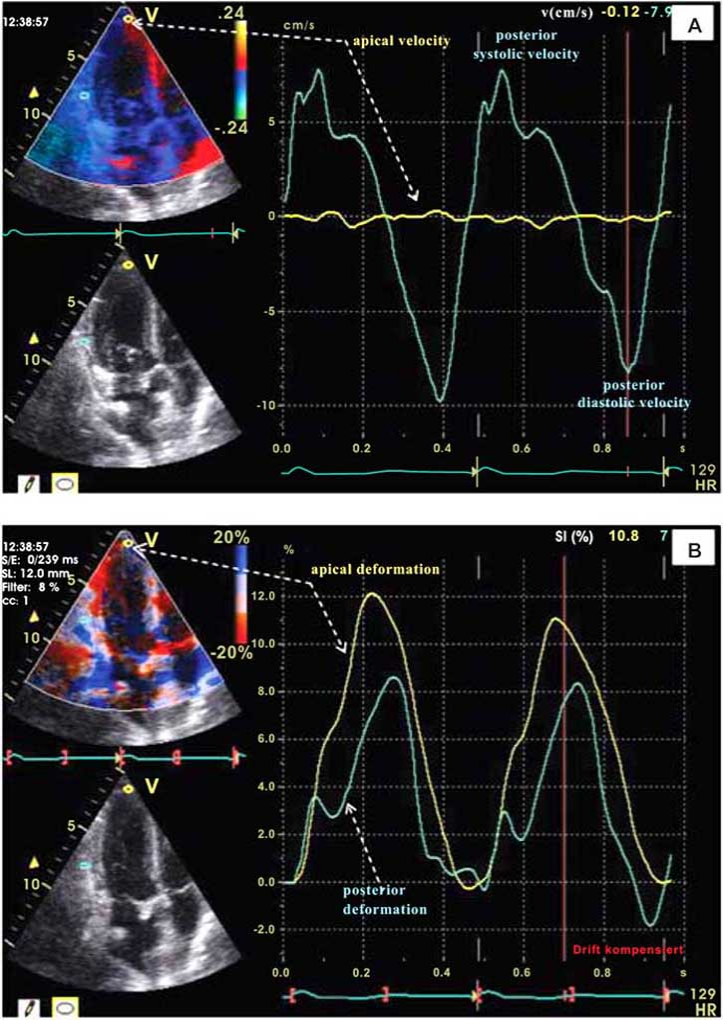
Tissue Doppler derived left ventricular wall motion velocity (panel A) and myocardial strain (panel B) images from a heart transplanted patient with normal cardiac function and no evidence of coronary artery disease. The velocity and strain curves were obtained in apical long axes views during the same cardiac cycle from the same two myocardial regions (posterior-basal and apical). Because of the velocity gradient which normally exists between the basal and apical LV regions (highest at the base and lowest in apical regions), the assessment of wall motion velocity is not useful for detection of regional differences in contractile function. Thus, as shown by the yellow curves, despite the very low wall motion velocity in the apical region (panel A), the longitudinal myocardial shortening in this region can be even higher than in basal regions (panel B).
